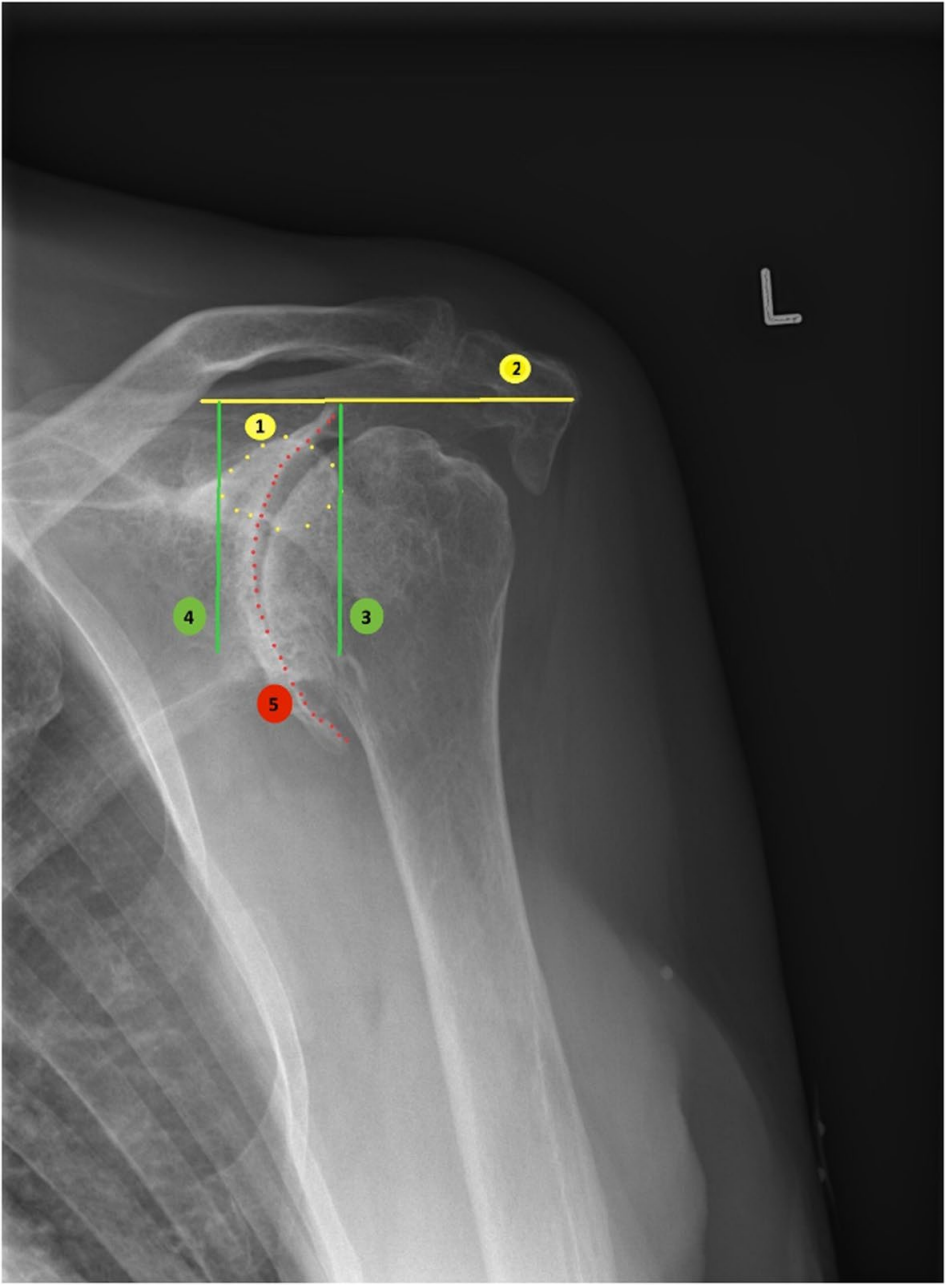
Assessment of medialization on true AP radiograph
